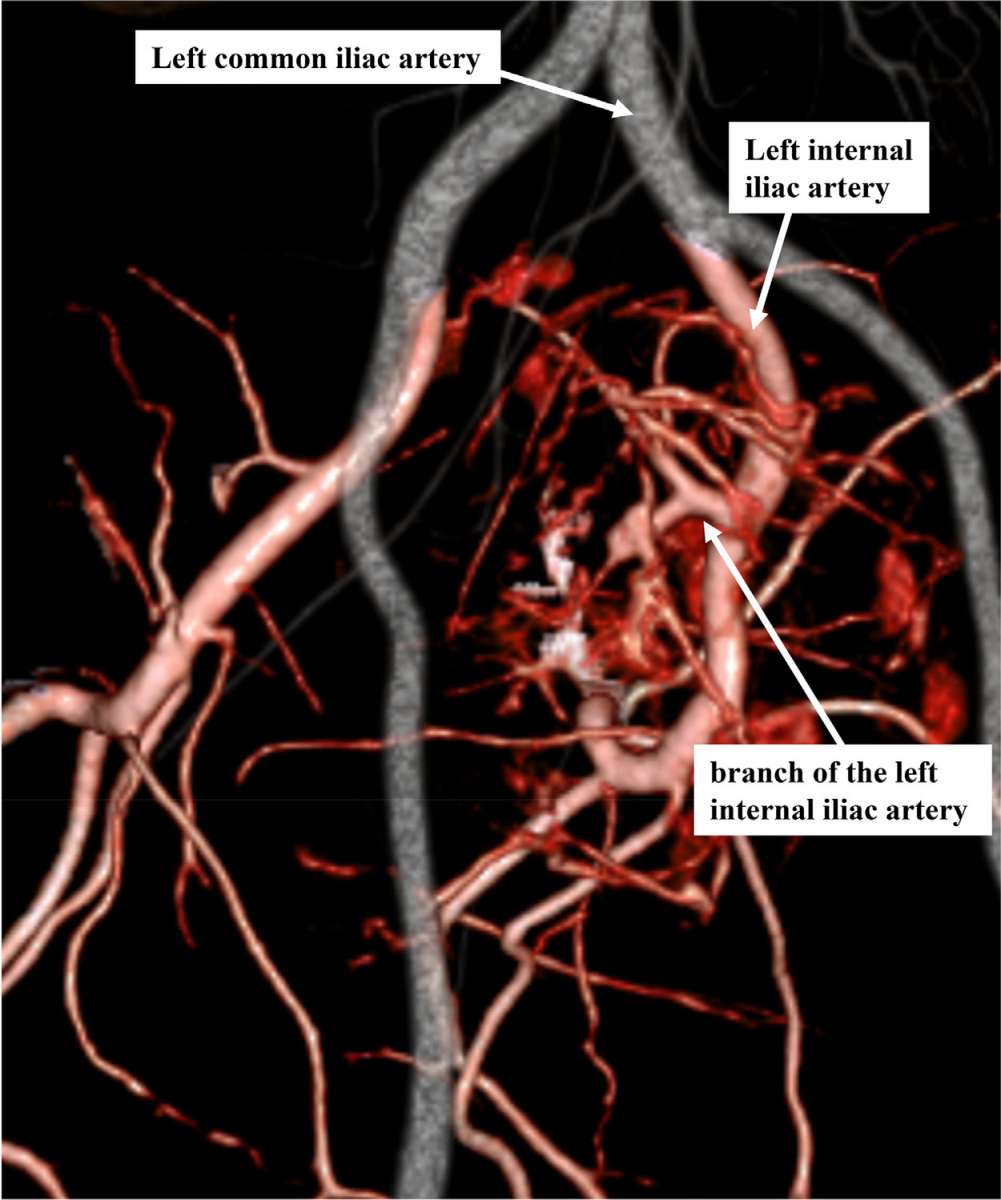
Contrast-enhanced CT 3D vascular reconstruction image of the common iliac artery region. The tumor was highly perfused, and the main feeding artery was a branch of the left internal iliac artery.
